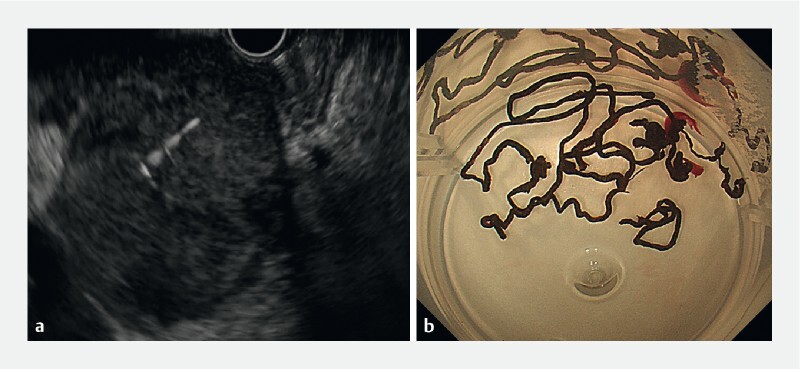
Endoscopic ultrasonography showed a low-density round lesion measuring 4.5 × 5.1 cm with regular borders in the head of the pancreas.
